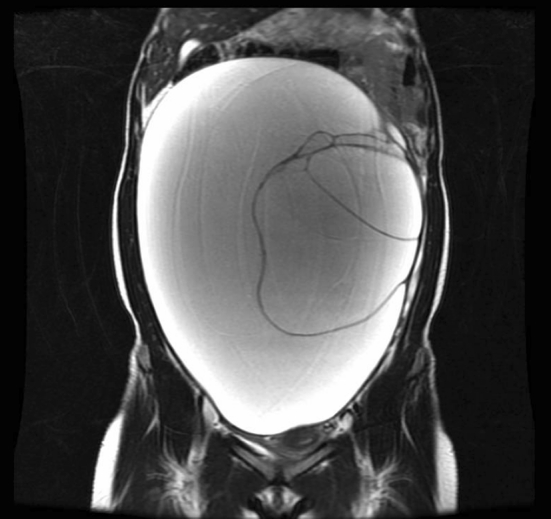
Patient 1: MRI (T2WI) showed a large multiocular ovarian cyst
